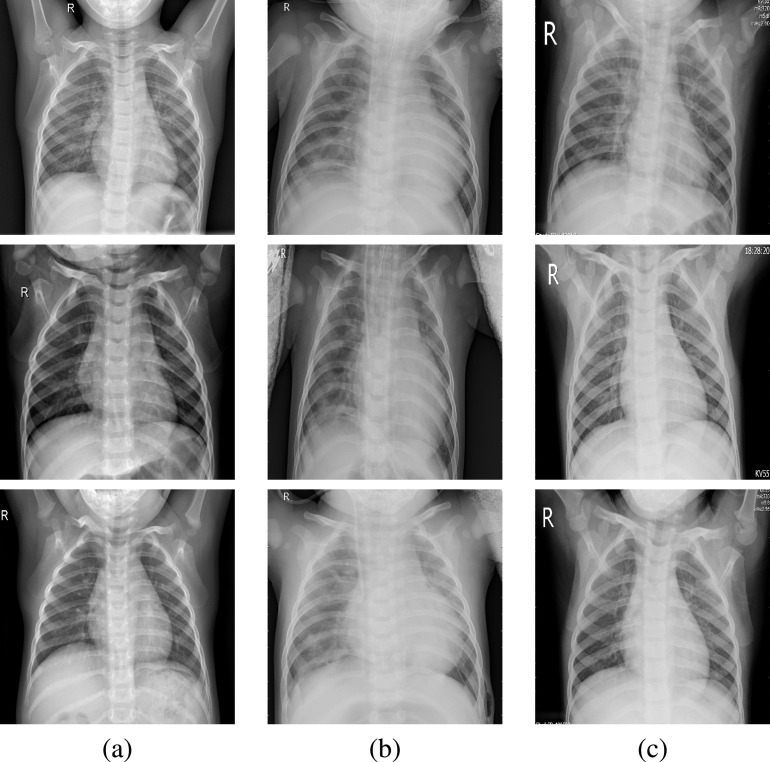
Samples of the original chest X-ray images [30]; (a) Normal cases, (b) Bacteria cases, and (c) Viral cases.
